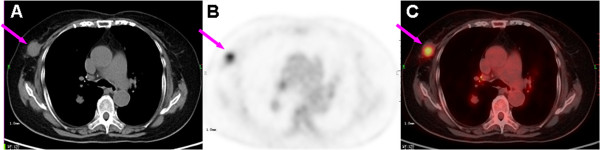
Computed tomography (CT) (A),^18^F-fluoro-deoxyglucose positron emission tomography (FDG-PET) (B), and fused PET/CT (C) images of the mammary lesion. Axial images show a 2.7×2.5cm, well-circumscribed, soft-tissue nodule with obvious FDG uptake (SUVmax of 7.30) in the right breast (arrows).
